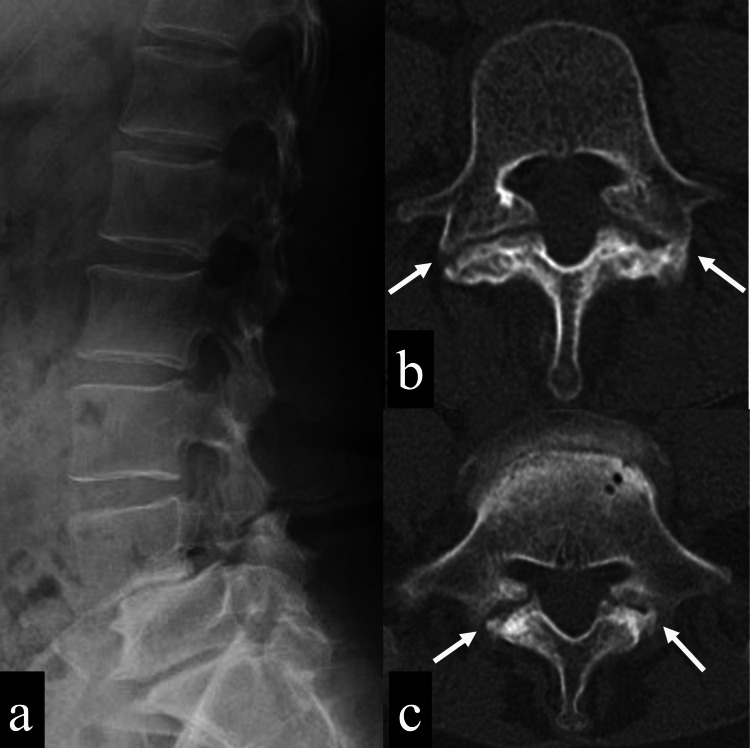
Preoperative imaging 1a: A plain lateral X-ray image of the lumbosacral spine shows L4 isthmic spondylolisthesis with Meyerding classification grade 2 anterior slip and L5 spondylolysis without slip. 1b: A plain CT axial view (L4 level) shows pseudoarthrotic isthmic spondylolisthesis at the L4 (white arrows). 1c: A plain CT axial view (L5 level) shows pseudoarthrotic spondylolysis at the L5 (white arrows).
